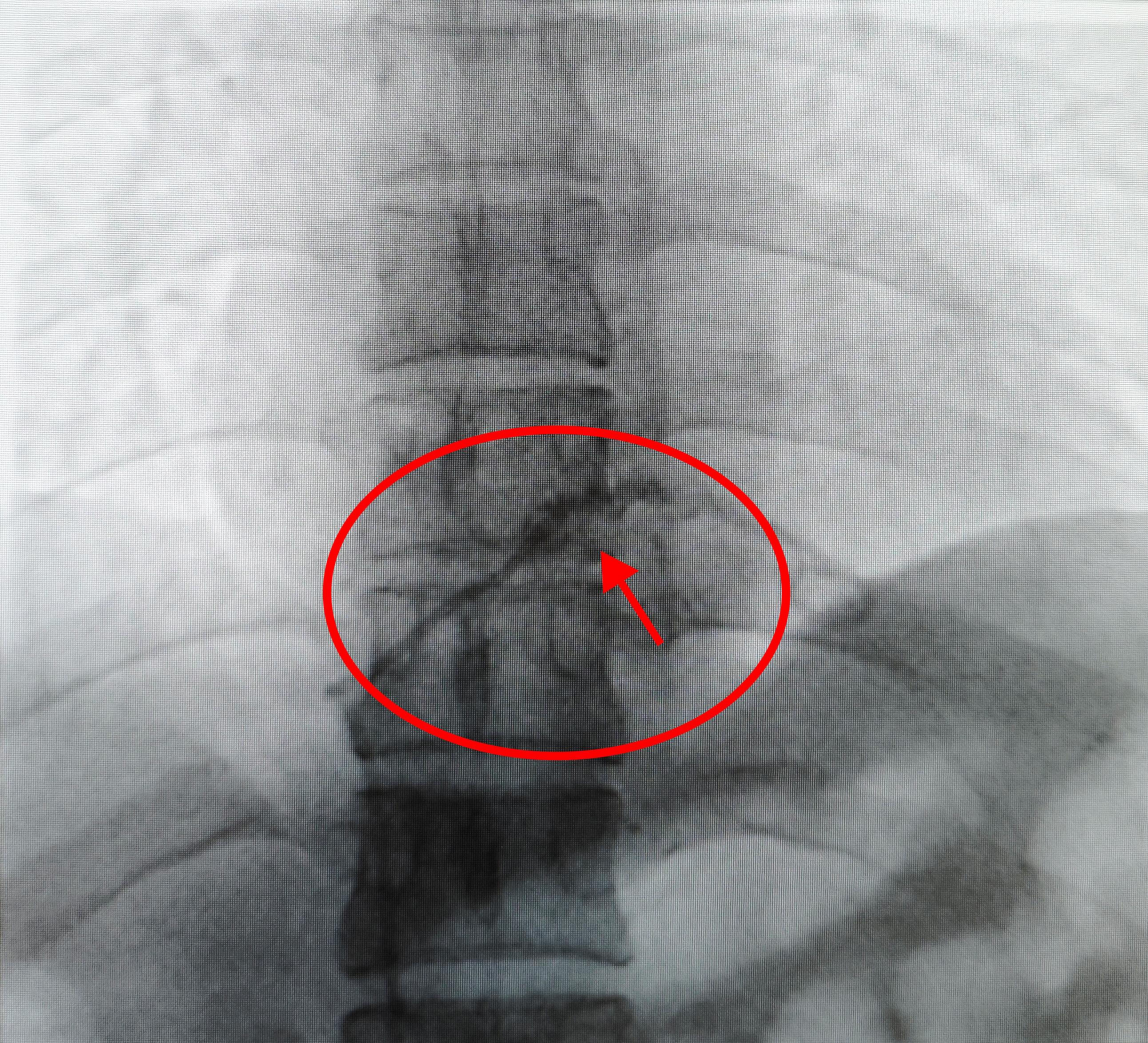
The chest CT report outside the hospital showed that after PICC placement, one of the PICC tips was located in the pulmonary artery corresponding to the dorsal segment of the left lower lobe; and the right atrium and right ventricle appeared to have the shadow of the winding pipe, and the superior vena cava was not clearly visible.
